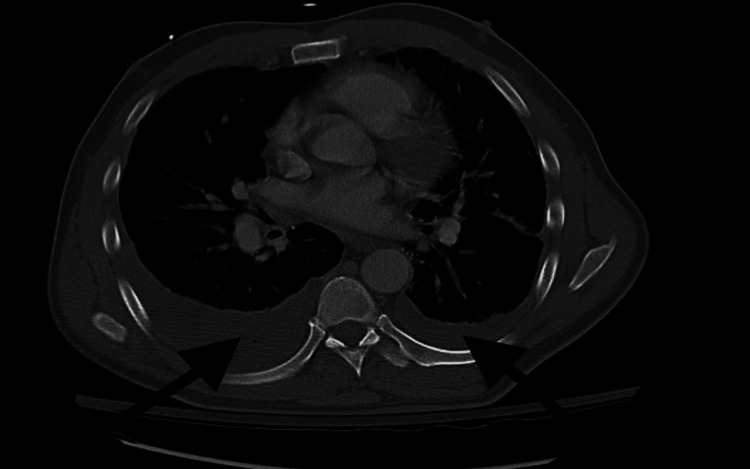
CT chest demonstrating bilateral pleural effusions (arrows), with the right side more prominent. These findings, along with mediastinal lymphadenopathy, raised concern for a potential underlying infectious, inflammatory, or neoplastic etiology.
